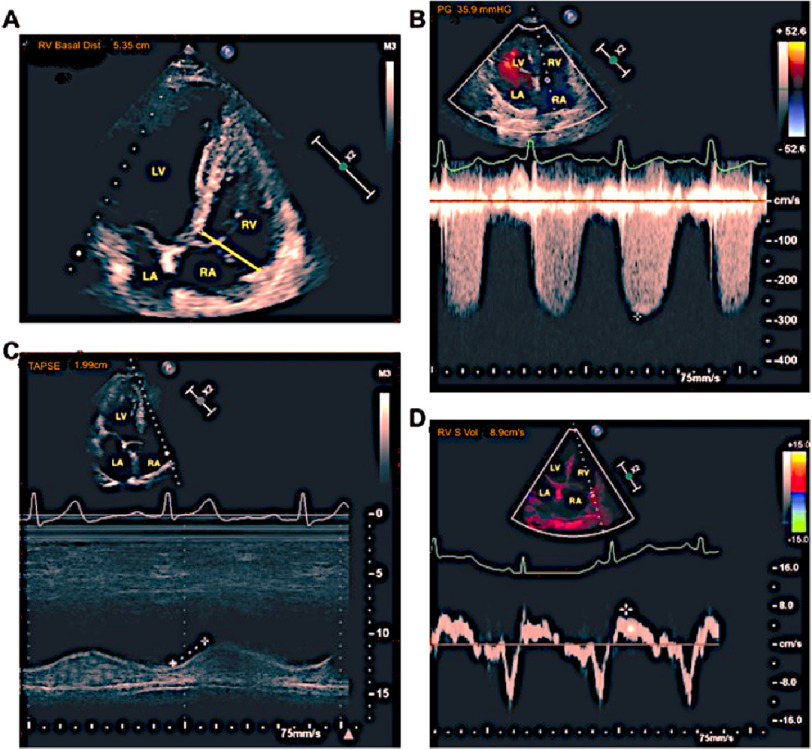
Two-dimensional transthoracic echocardiography of a patient with right ventricular dysfunction with serious SARS-CoV-2 delta strain patients. (A) Enlarged right ventricle (RV). (B) Increased systolic pulmonary artery pressure (sPAP). (C) Reduced tricuspid annulus plane systolic excursion (TAPSE). (D) Decreased TDI-derived tricuspid lateral annular systolic velocity (*S*′).
